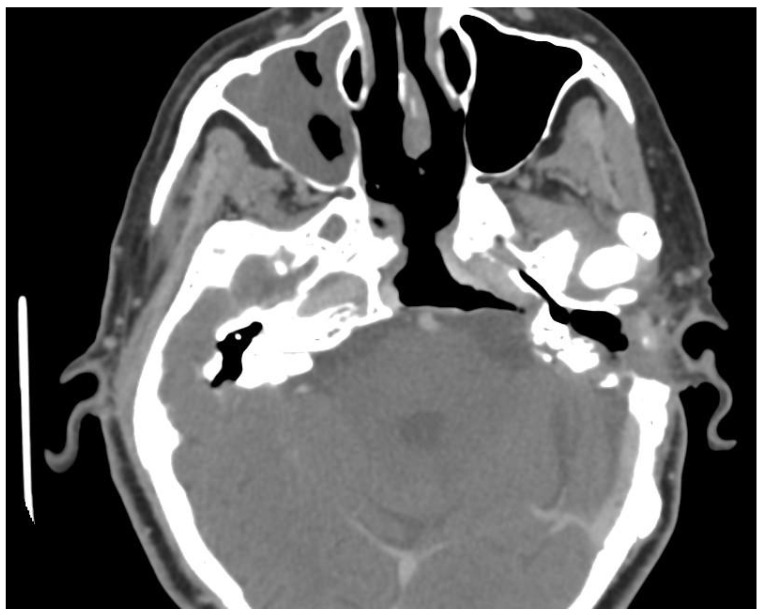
Postoperative CT imaging of the skull base, with no detectable recurrence.
